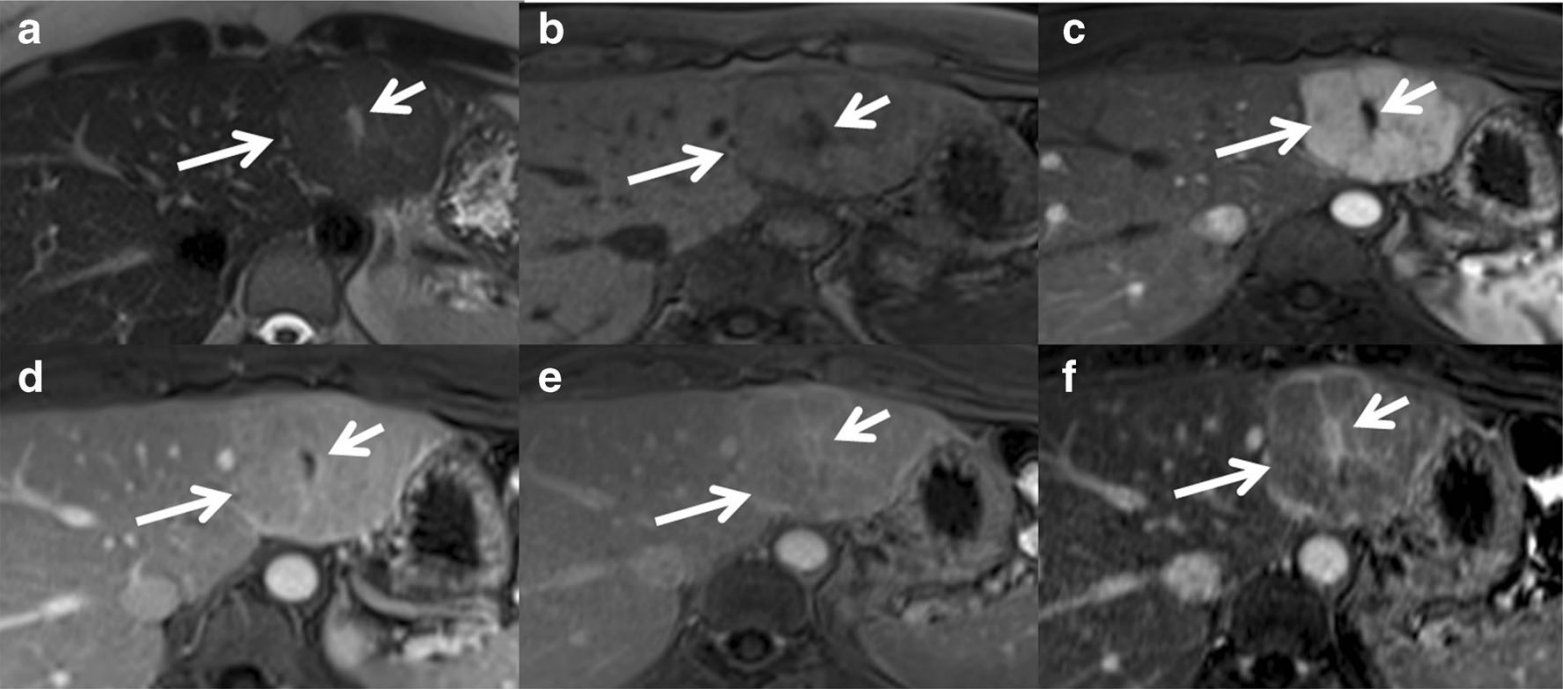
Classic focal nodular hyperplasia (FNH) in a 40-year-old female incidentally detected on ultrasound (not shown here). (a) Axial T2W MRI shows a well-circumscribed T2 isointense lesion (*long arrow*) in the left lobe of the liver with a central T2 hyperintense scar (short arrow). (b) Axial T1W MRI shows a well-circumscribed T1 isointense lesion (long arrow) in the left lobe of the liver with a central T2 hypointense scar (*short arrow*). (c) Axial post-contrast MRI in the arterial phase reveals intense enhancement of the lesion (*long arrow*) with a non-enhancing central scar (*short arrow*). Axial post-contrast MRI in the venous (d) and delayed phases (e and f) shows the isointense nature of the lesion (*long arrow*) with progressive delayed enhancement of the central scar (*short arrow*)
